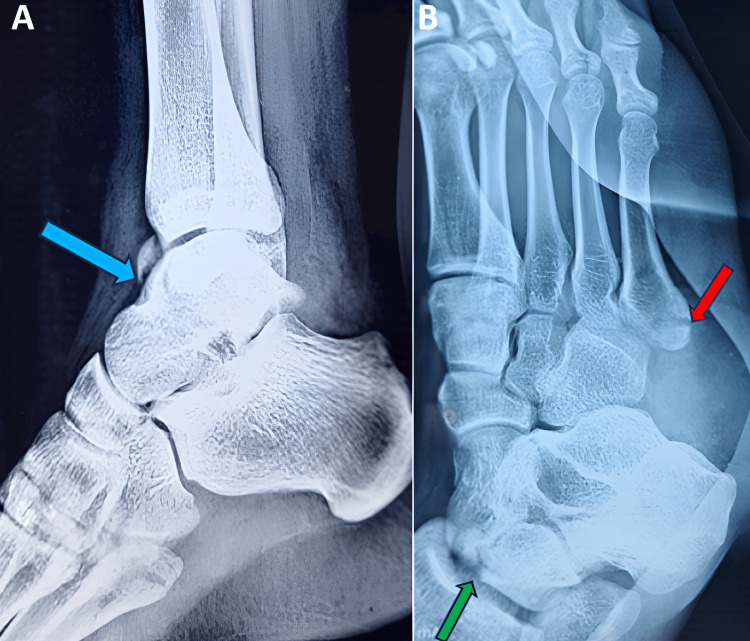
X-ray images of the right ankle and right foot. The images show (A) lateral view of the right ankle showing talus fracture (blue arrow) and (B) oblique view of the right foot showing fifth metatarsal base (zone 1) fracture (red arrow) and talus fracture (green arrow).
